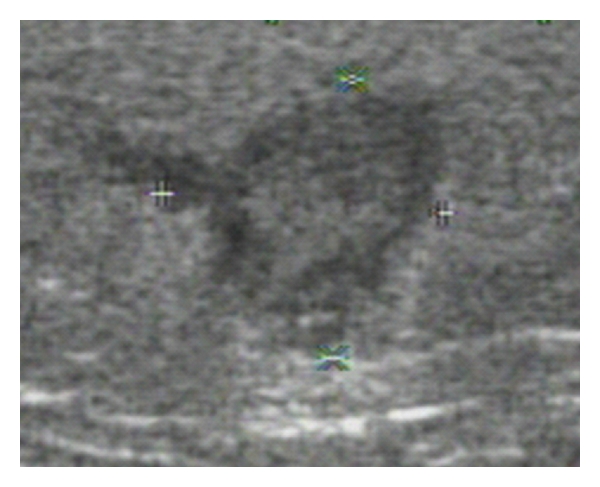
A subcutaneous nodule was detected by sonogram. Size: 10.3 × 10 mm. Depth: 11.3 mm.
